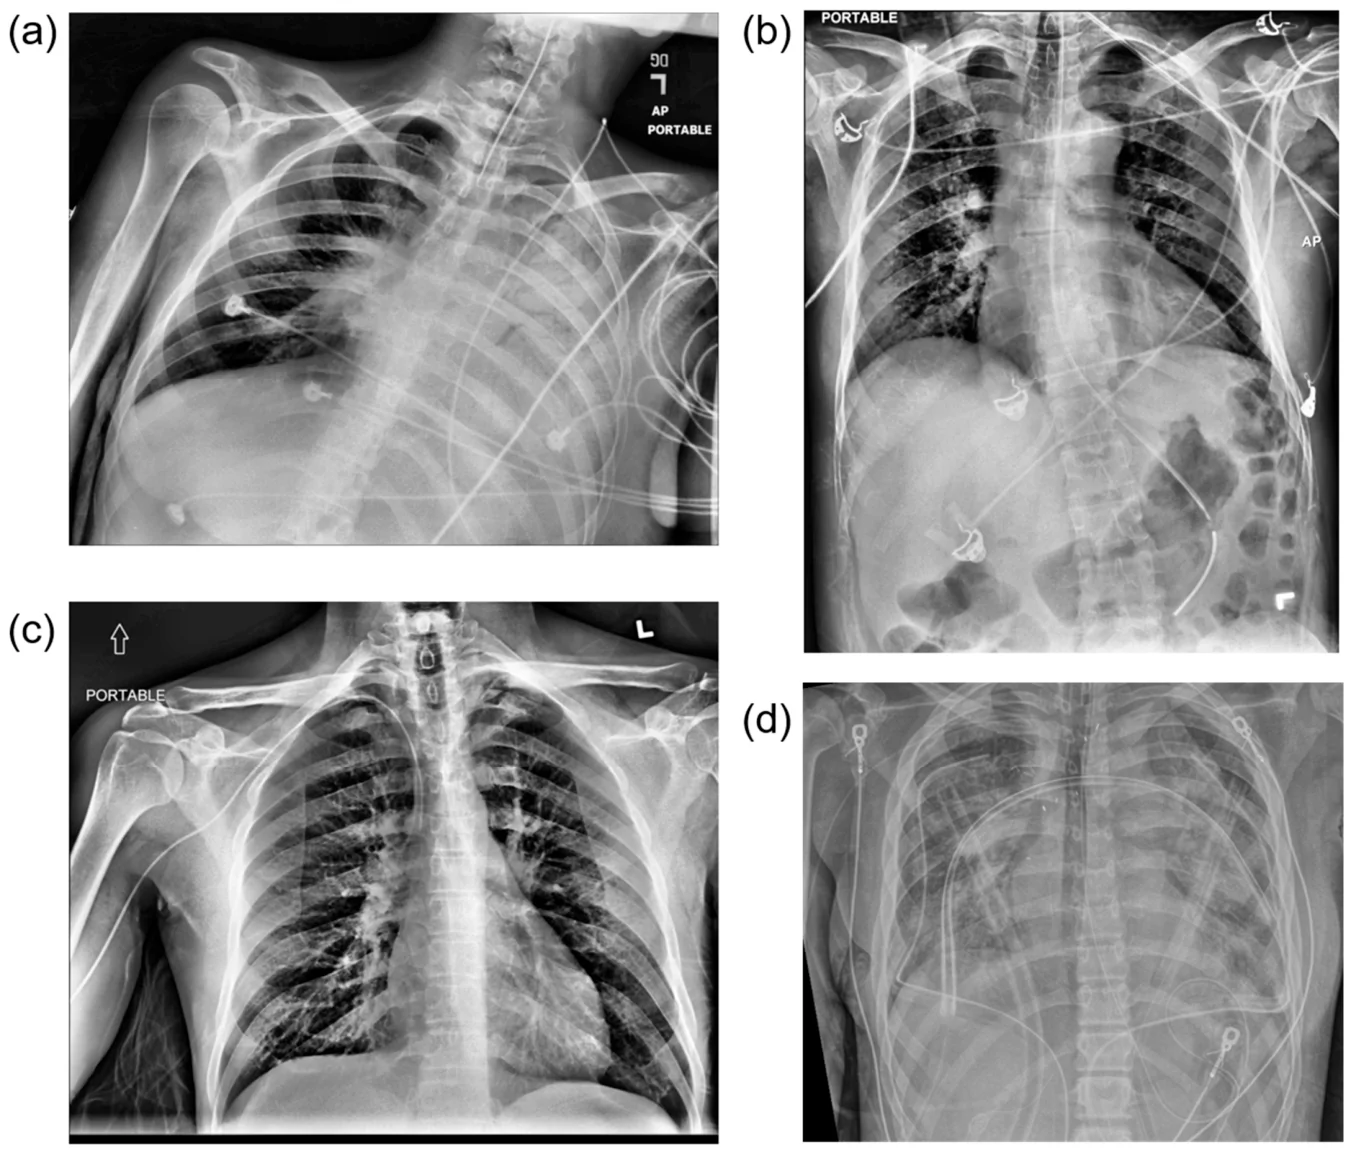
Chest radiographs demonstrating the four primary categories of line, drain, and airway (LDA) devices. Images have been contrast-enhanced to improve visualization of device trajectories and distal tips. (a) Artificial Airway: Frontal chest radiograph demonstrating an endotracheal tube (ETT) with the tip in the mid-trachea above the carina. (b) Enteric Tube: Frontal chest radiograph demonstrating a nasogastric tube (NGT) coursing into the stomach with the tip in the mid-stomach. (c) Central Venous Catheter: Frontal chest radiograph demonstrating a peripherally inserted central catheter (PICC) with the tip in the lower third of the superior vena cava. (d) Chest Tube: Frontal chest radiograph demonstrating four chest tubes, two within each hemithorax.
